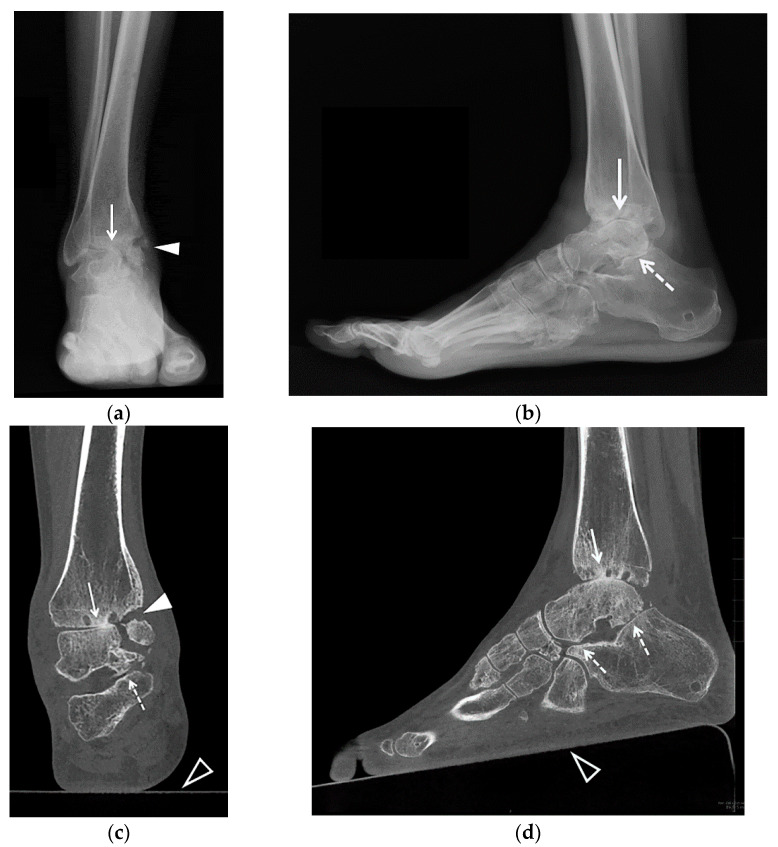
(a–d). A 25-year-old female with posttraumatic osteoarthritis of the right tibiotalar and subtalar joints. AP (a) and lateral (b) weight bearing radiographs and coronal (c) and sagittal (d) reformatted weight bearing CT images of the right ankle show an ununited medial malleolus fracture (arrowheads) and severe osteoarthritis of the tibiotalar (arrows) and subtalar joints (dashed arrows) with asymmetric joint space narrowing, subchondral sclerosis and small cyst-like changes. In (b) note the external fixator pin track in the calcaneal tuberosity. In (c,d) note a platform underneath of the foot (open arrowheads) with the CT images acquired in a cone-beam CT scanner dedicated to extremity imaging that allows the assessment of the alignment. Case courtesy of Imran Omar MD, Chicago, IL.
